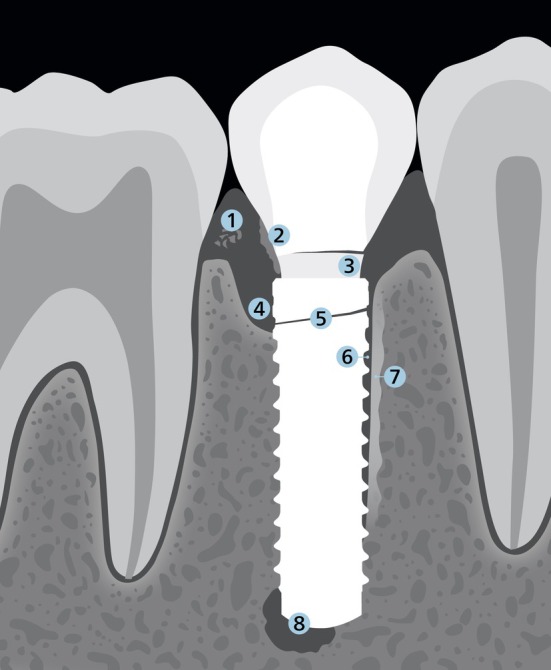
Radiographic findings of interest: (1) residual bone graft substitute particles in the peri‐implant soft tissues, (2) residual cement, (3) misfit of the implant‐supported prosthesis, (4) crestal bone loss, (5) implant fracture, (6) peri‐implant sheathing, (7) peri‐implant corticalization, and (8) retrograde peri‐implantitis.
